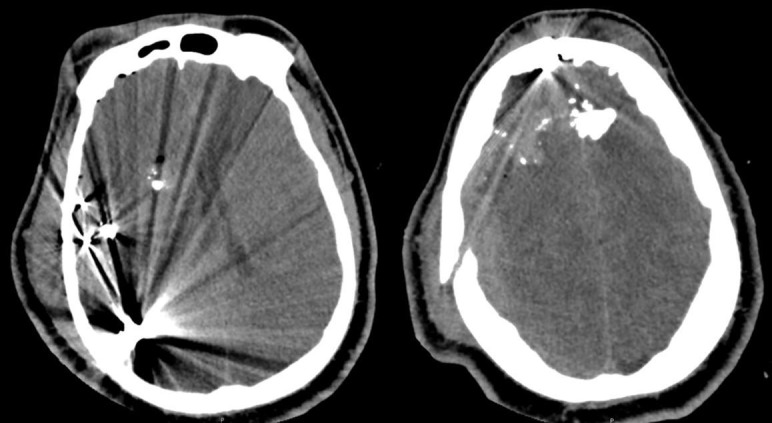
Presenting computed tomography (CT) head which revealed severe intracranial injury from a high-velocity projectile with a trajectory from the right parietal lobe through the left frontal lobe with contusions, ballistic and skull fragments along the pathway. Additional findings included a 7 mm right sided subdural hematoma and a right-to-left midline shift of 5.5 mm.
